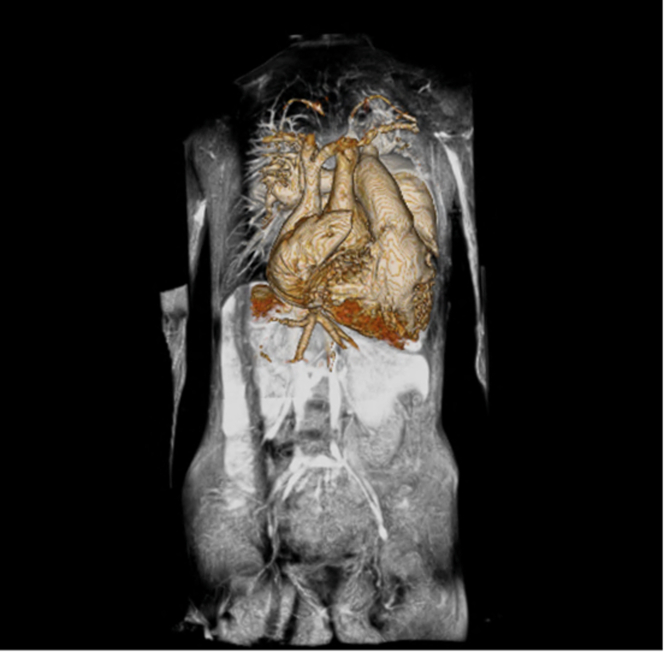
Initial Magnetic Resonance Imaging Findings Magnetic resonance imaging demonstrated the sheer amount of space within the thorax taken up by the patient's large heart and great vessels.
